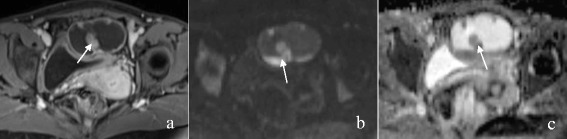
A 53-year-old woman with left-sided OEC. Contrast-enhanced T1WI with fat suppression (a) demonstrates a mainly cystic mass with mural nodules (arrow), which show a marked enhancement (a) and a hyperintensity on DWI (b) with an ADC value of 1.174 × 10^−3^ mm^2^/s(c).
